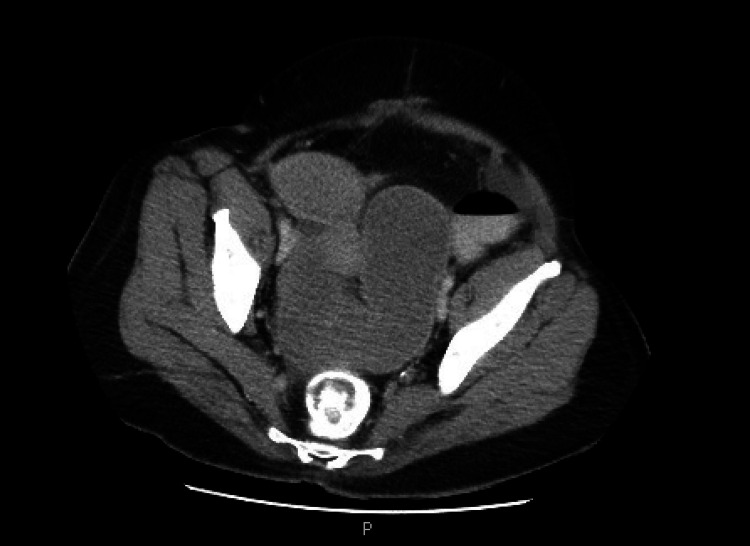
Computed tomography of the abdomen showing worsening high-grade small bowel obstruction
